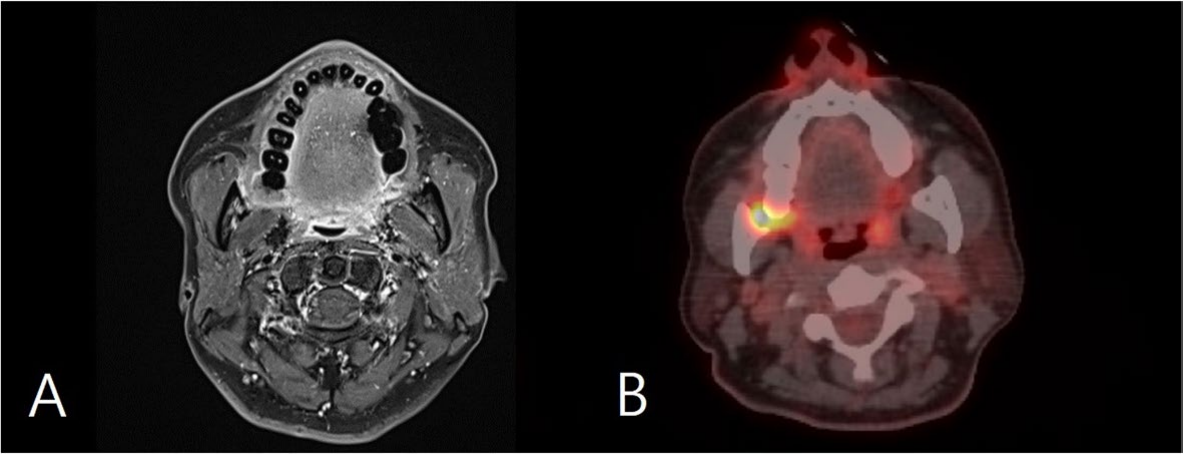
MRI and PET-CT showed irregular lesion of the right retromolar trigone region
